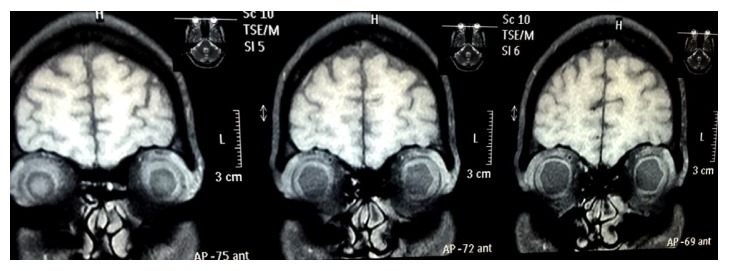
Coronal T1 weighted MRI of head and orbit showing bilateral choroidal detachment with bilateral suprachoroidal haemorrhage.
